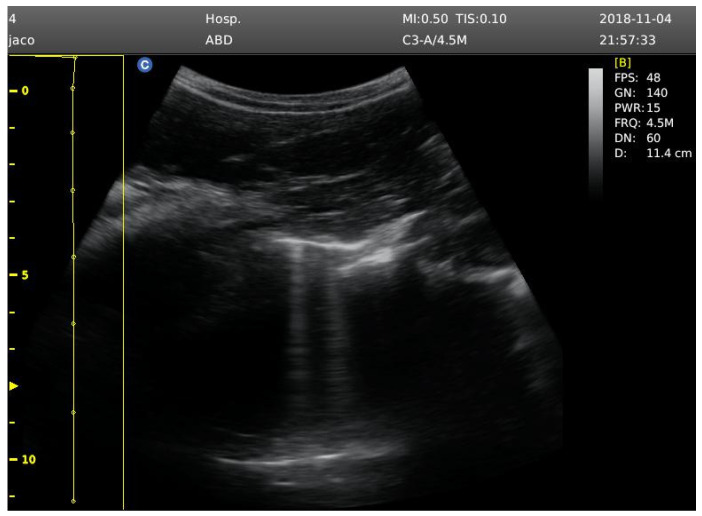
Signs of ultrasound B-lines. Image analyzed at 12 cm depth.
